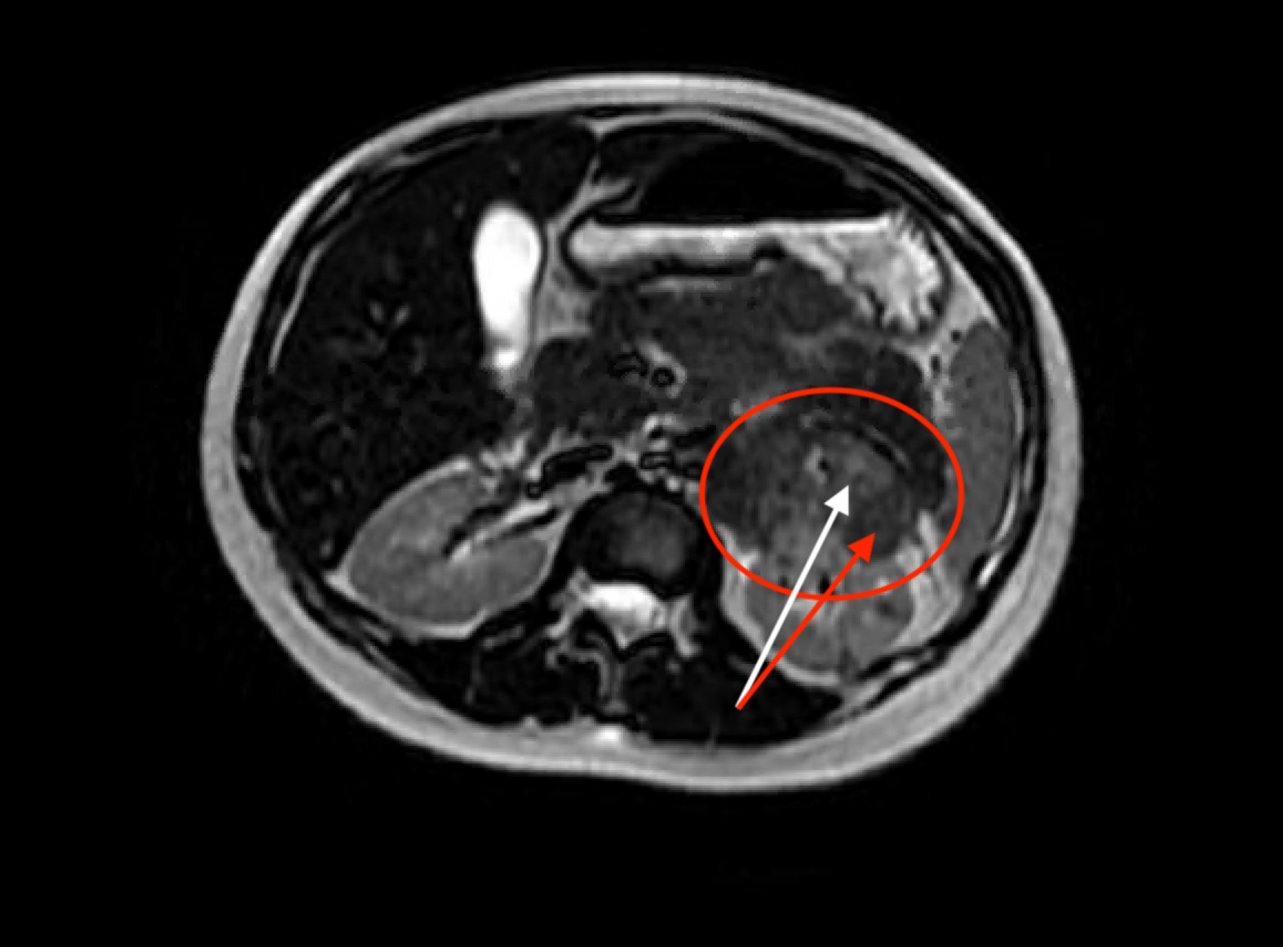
MRI of the abdomen without contrast showing a large heterogeneous mass arising from the superior aspect of the left kidney (red circle). Dense area (white arrow) contrasting with hypodense area (red arrow) suggesting the presence of fat and hypervascularity, indicative of angiomyolipoma.
